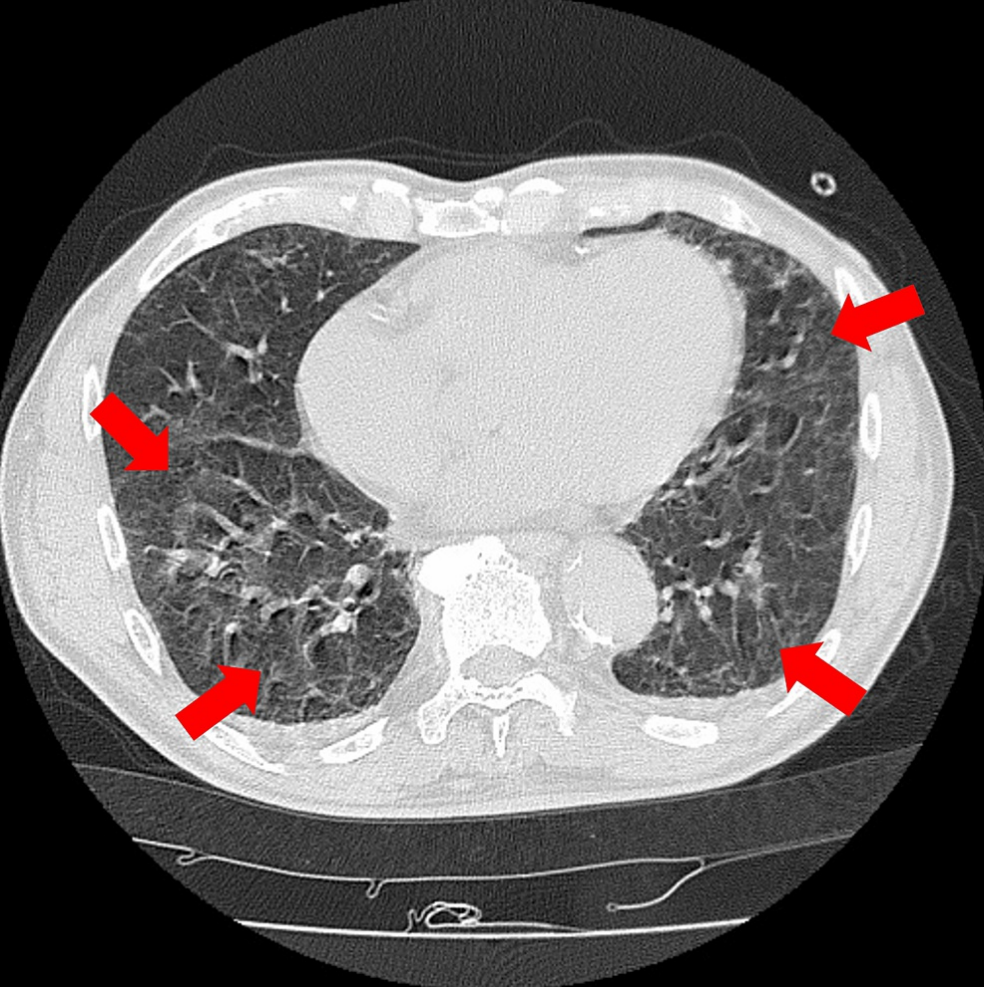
Mosaic attenuation in both lungs on a plain thoracic CT at the Th10 level (arrow)
